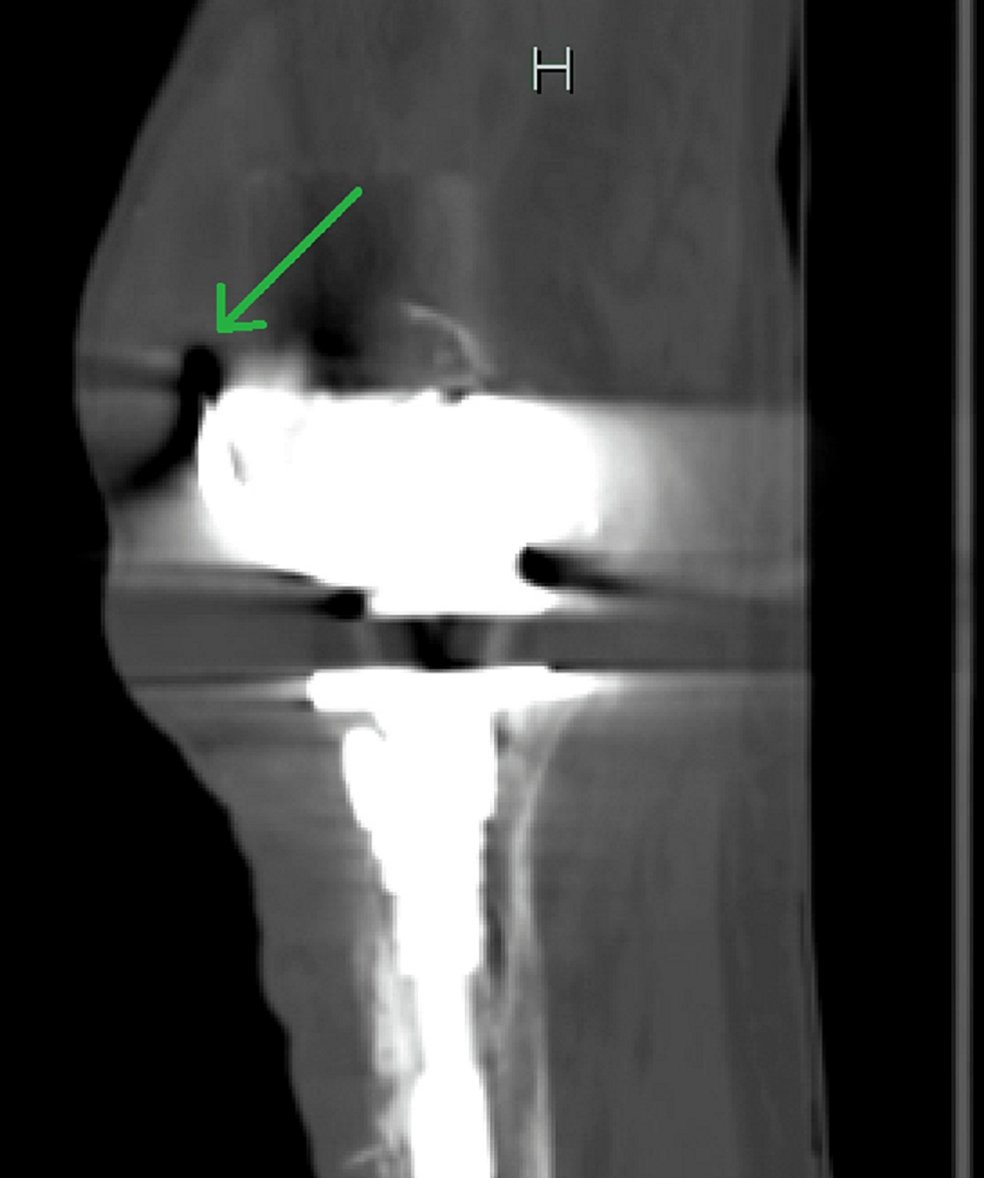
CT of the left lower extremity, sagittal cut, showing gas bubbles (green arrow) in the fluid collection, suggestive of an abscess. CT, computed tomography
